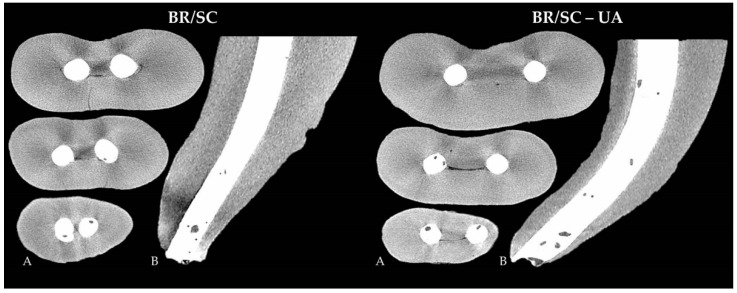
Cross-sectional images at the level of 5 mm, 3 mm and 1 mm from the apex (A) and longitudinal images (B), representing the quality and homogeneity of BR/SC (BioRoot RCS/single cone) and BR/SC-UA (BioRoot RCS/single cone with ultrasonic agitation) apical plugs.
